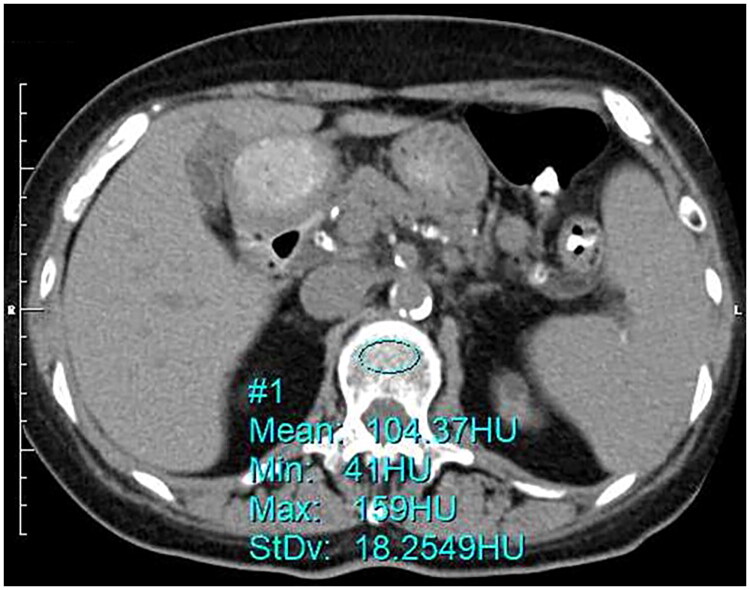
An example of measuring L1 CT-attenuation value. A single axial computed tomography (CT) slice of the first lumbar (L1) vertebral body was selected at the pedicular level. The region of interest (ROI) tool was used. The ROI was set to the maximum possible elliptical diameter without including the vertebral cortex or the basivertebral venous plexus. The average CT value within the ROI was defined as L1 CT-attenuation. In this case, the L1 CT-attenuation was 104.37 HU.
